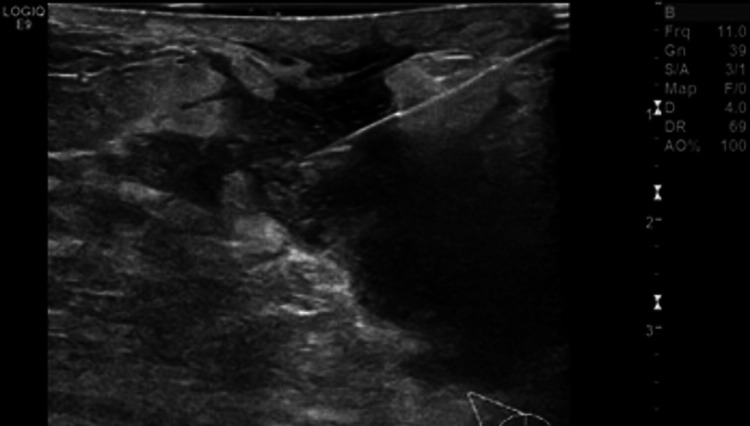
Ultrasound-guided aspiration of the fluid collection using 18G needle.
